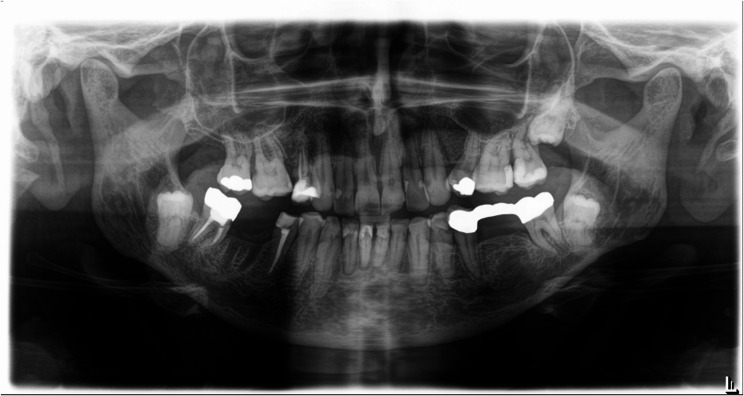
Panoramic radiograph of the same patient 5 years after radiotherapy with new restorations especially in the lower front and upper front teeth as well as a fresh alveolar wound after extraction of tooth 46
